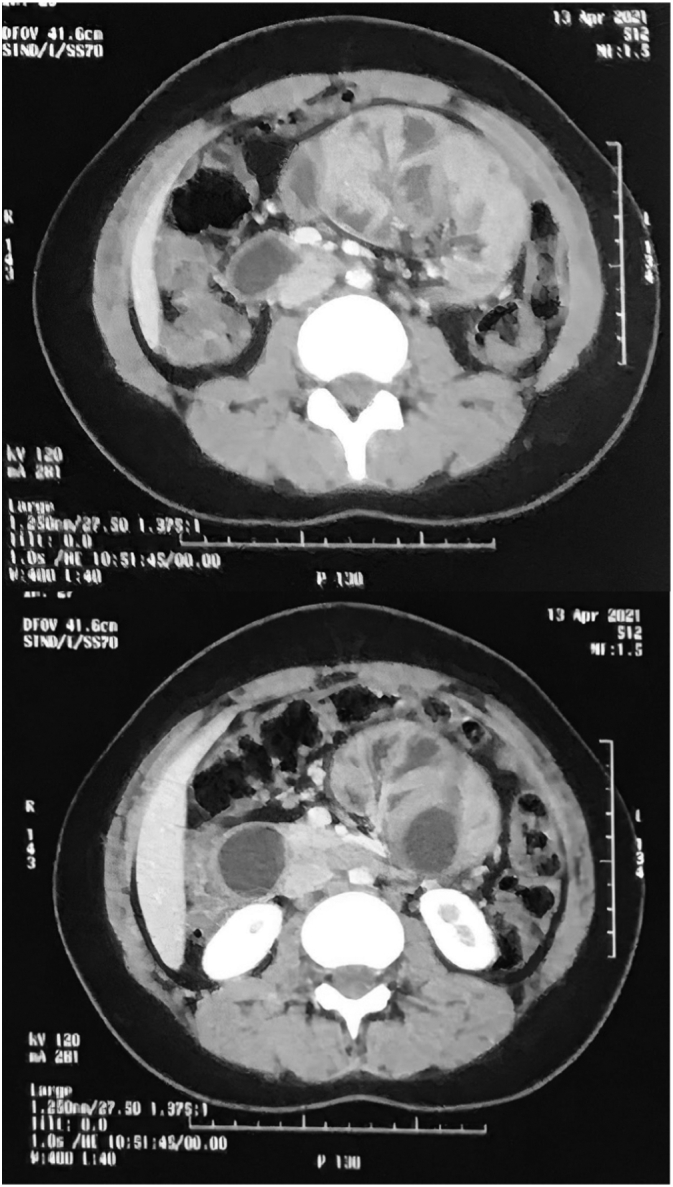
A CT scan showing dilated loops of small bowel.
